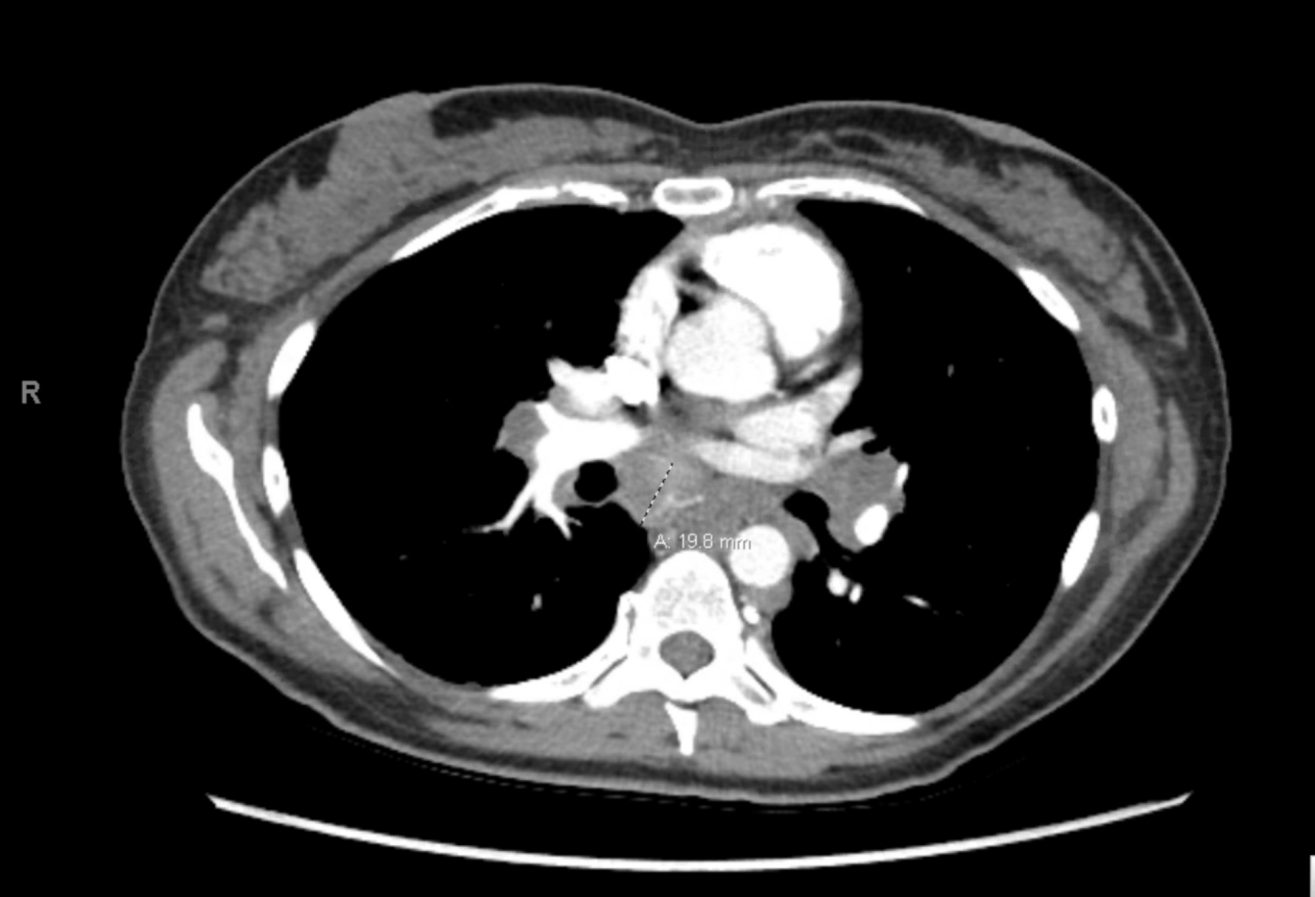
CT of the chest revealing mediastinal and hilar lymphadenopathy suspicious for sarcoidosis
